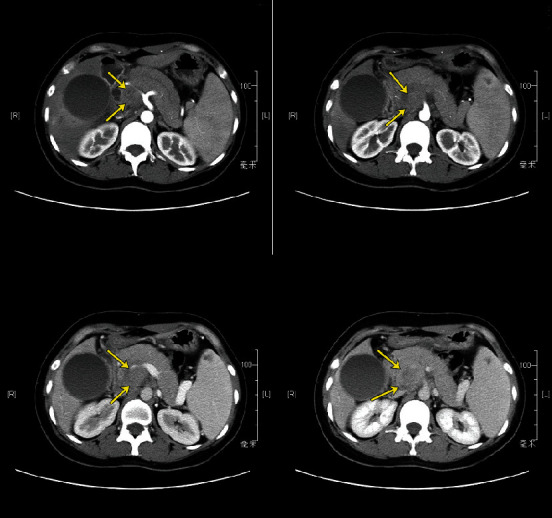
Indeterminate imaging. CT shows a diffusely enlarged gland with a low-density mass (arrows marked) adhering tightly to the superior mesenteric artery (SMA) and invading the superior mesenteric vein/portal vein (SMV/PV).
